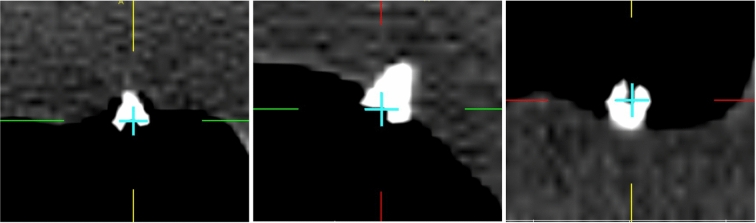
Partial screenshot of the intraoperative navigation while the accuracy of the registration on a target was qualitatively evaluated by localizing the implanted titanium screws with the navigation probe. The crosshair on DICOM views (axial, sagittal and coronal, from left to right) indicates the actual position of the probe tip placed on the screw head. Small titanium screws are known to hardly produce image reconstruction artefacts in CT imaging [30]; thus, the clarity of the images is not affected
